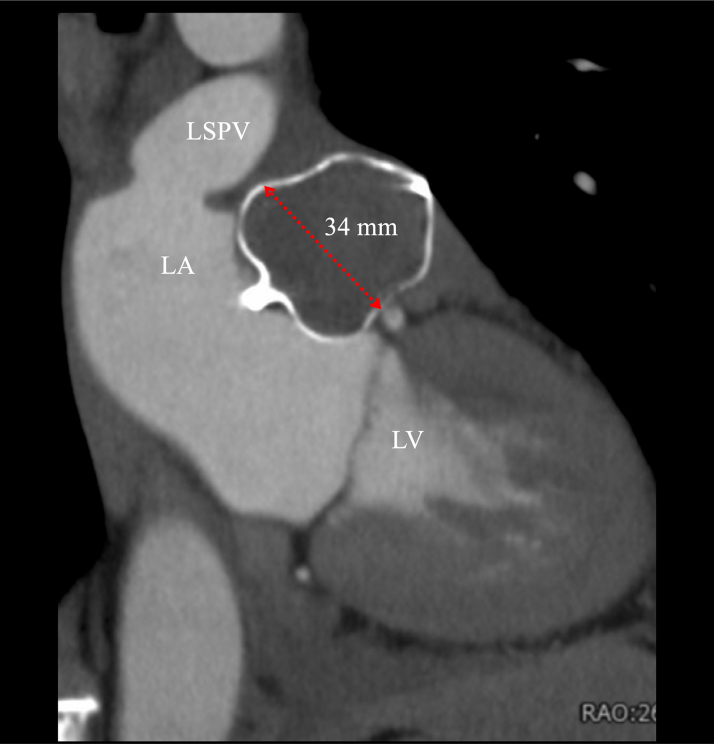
Follow-Up CT Findings Cardiac CT at 1-month follow-up showing stable device position, appropriate compression (15%), and no device-related thrombus. CT = computed tomography; LA = left atrium; LSPV = left superior pulmonary vein; LV = left ventricle.
